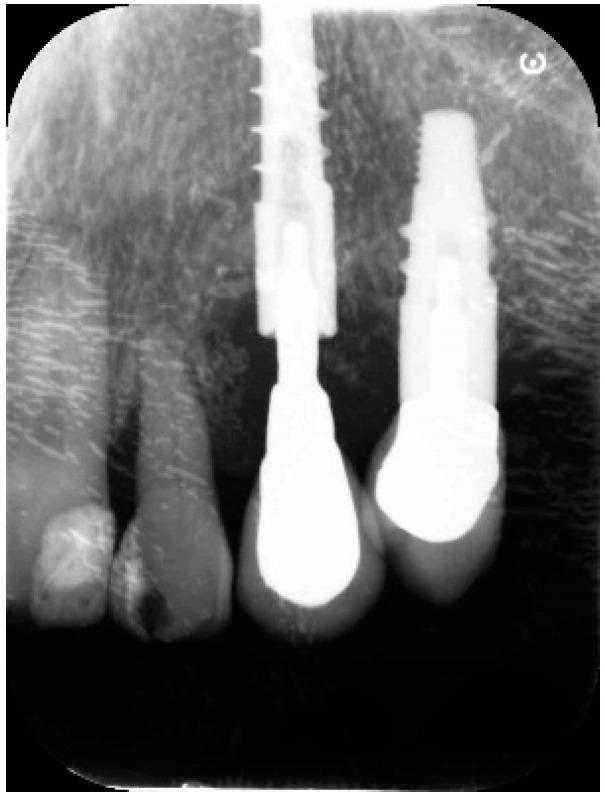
Endoral X-ray taken 3 months after treatment.
